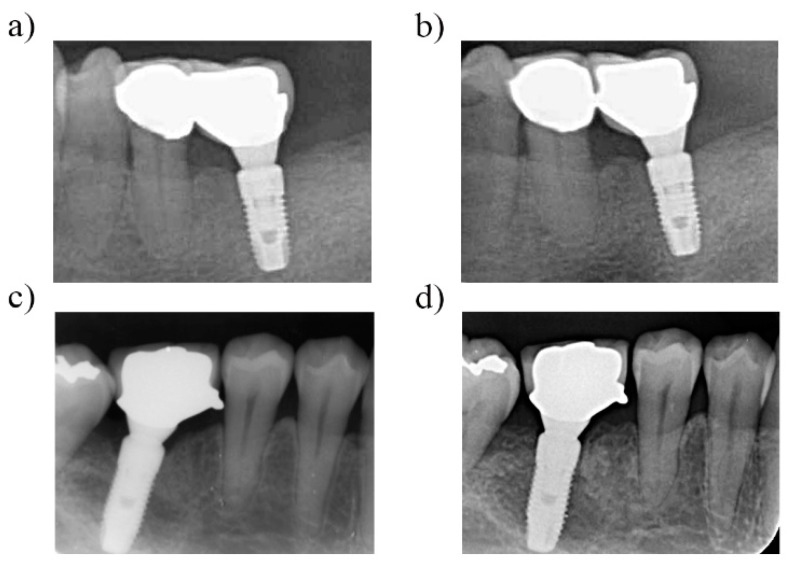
Radiographic follow-up images of dental implants. (a) Platform-switched dental implant in lower left area immediately after prosthetic delivery. (b) Radiographic view of the same dental implant in the lower left area at a three-year follow-up, with no apparent bone remodeling. (c) Platform-switched dental implant in the lower right area immediately after prosthetic delivery, (d) Radiographic view of the same dental implant in lower right area at three-year follow-up, with minimal bone remodeling.
